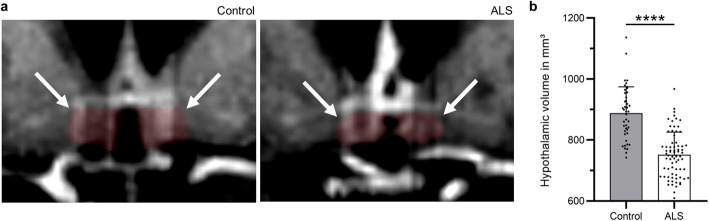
Hypothalamic atrophy in ALS patients. a Representative T1w MRI of hypothalami of a healthy control and an ALS patient; hypothalami are shadowed in red (arrows). b The hypothalamic volume was significantly decreased in ALS patients (*n* = 72) compared to the controls (*n* = 43). Bars represent mean ± SD. *P* < 0.000 1
